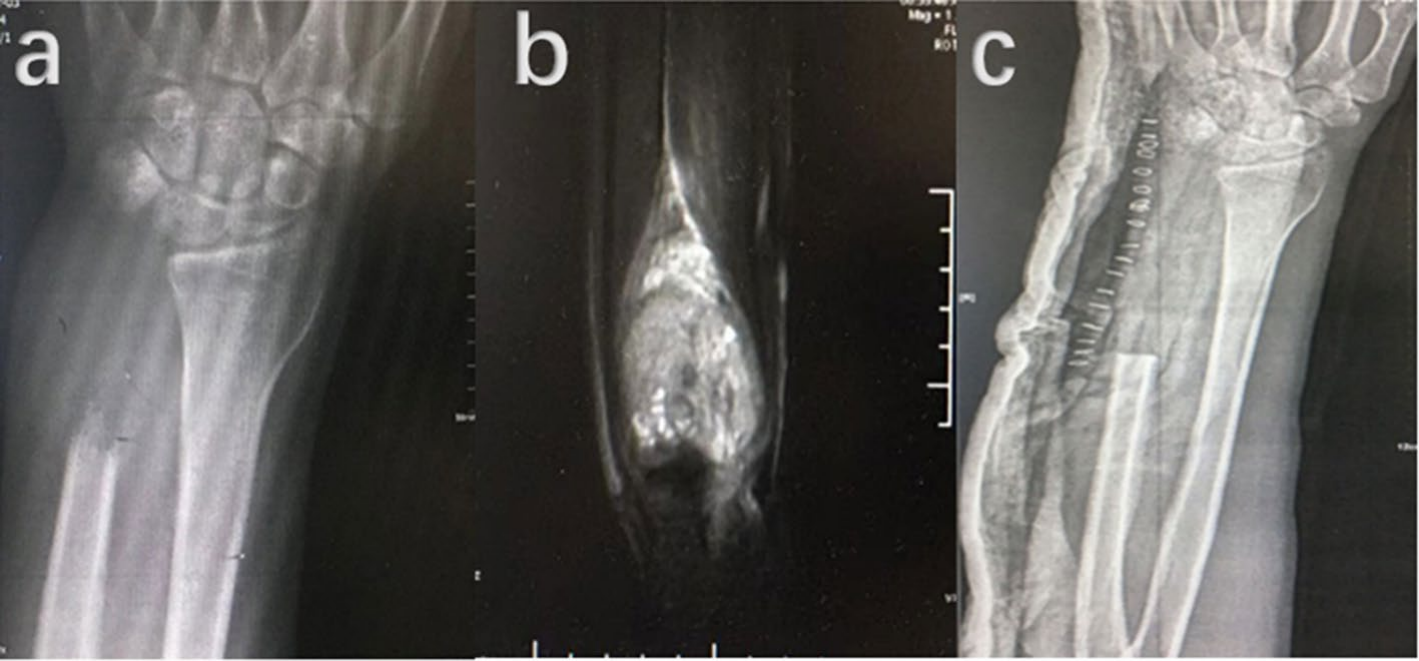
Radiographic images of a 24-year-old male patient with GCTB located in distal ulna at the time of primary surgery. a Plain radiography before operation showed osteolytic destruction at left distal ulna. b MRI revealed intermediate to high intensity signal in the lesion. c Post-operative plain radiography after distal ulna has been resected
